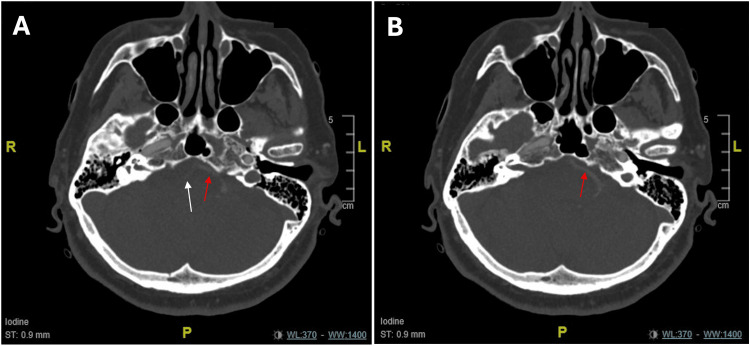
CT angiography of the head (A) The white arrow indicates a hypoplastic right vertebral artery with calcifications. The red arrow indicates the left vertebral artery with reduced contrast opacification compared with the internal carotid arteries and the middle cerebral arteries. (B) The red arrow shows reduced contrast opacification of the left vertebral artery when compared with the internal carotid arteries and middle cerebral arteries
